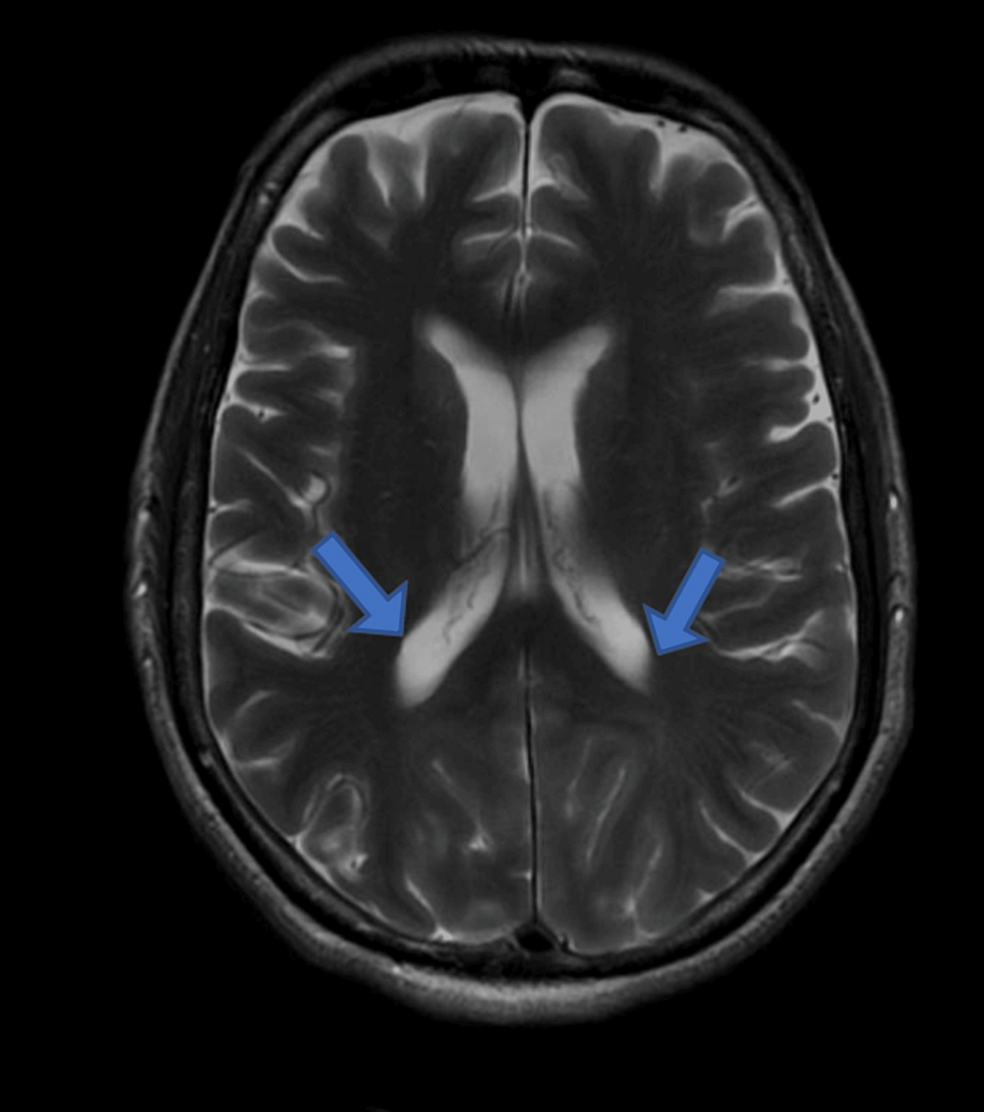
MRI brain coronal section showing hyperintensities in T2-weighted image in the bilateral parieto-occipital lobe extending in asymmetric fashion to the bilateral cerebellum, brainstem, and thalami predominantly involving the white matter
